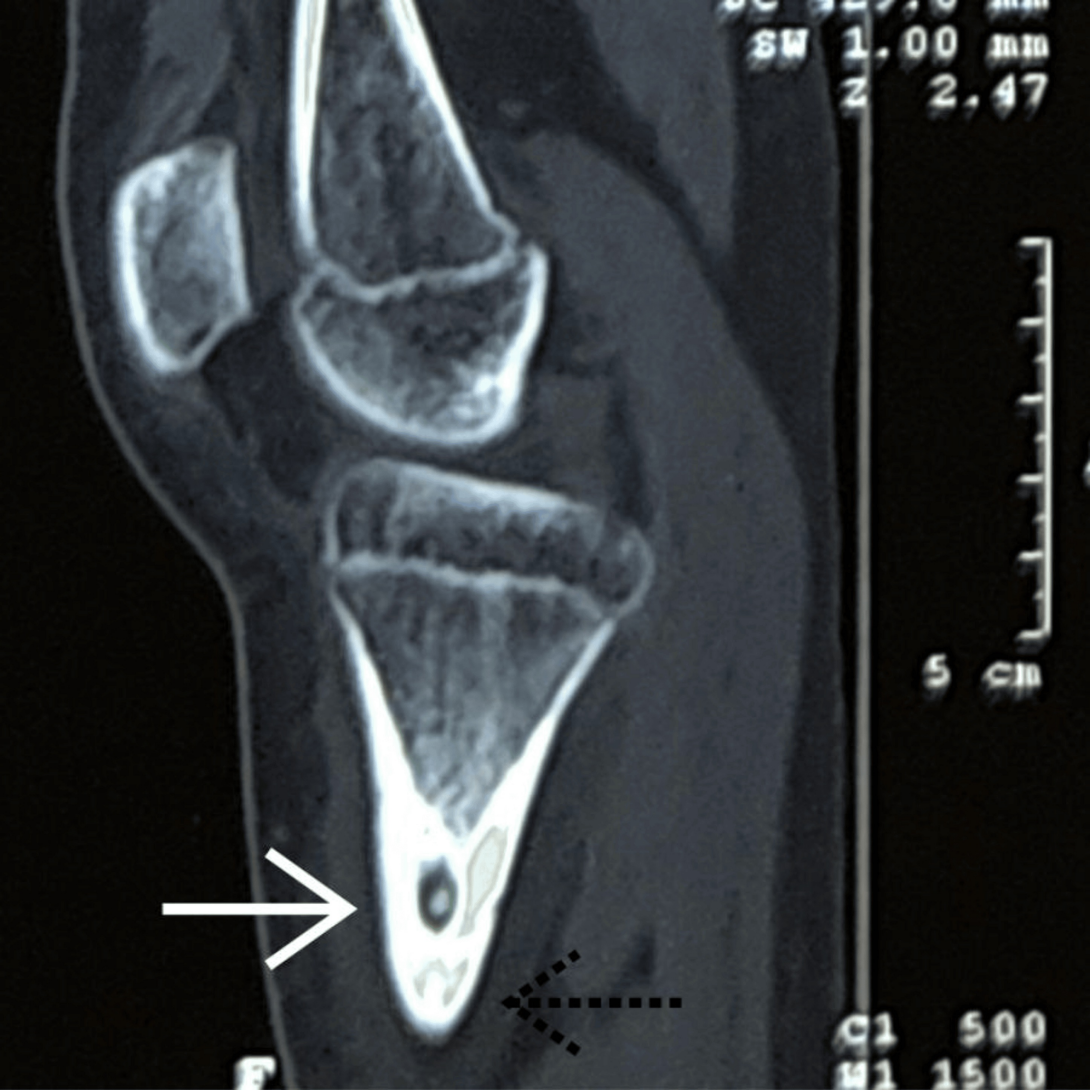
Plain CT of the left leg on the sagittal section showing an osteoid osteoma with two nidi Two well-defined sclerotic lesions with central lucency, the largest measuring 11 x 6 millimeters, at the proximal meta-diaphyseal junction of the left tibia, accompanied by adjacent cortical thickening.
